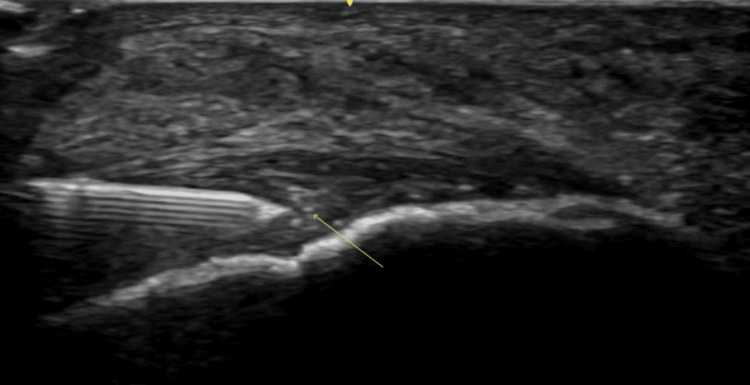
Ultrasound-guided injection of Achilles tendon Image of an ultrasound-guided injection of BTX-A using a long-axis, in-plane approach directed at the area of the most notable tendinopathic changes (arrow). BTX-A: botulinum toxin A
